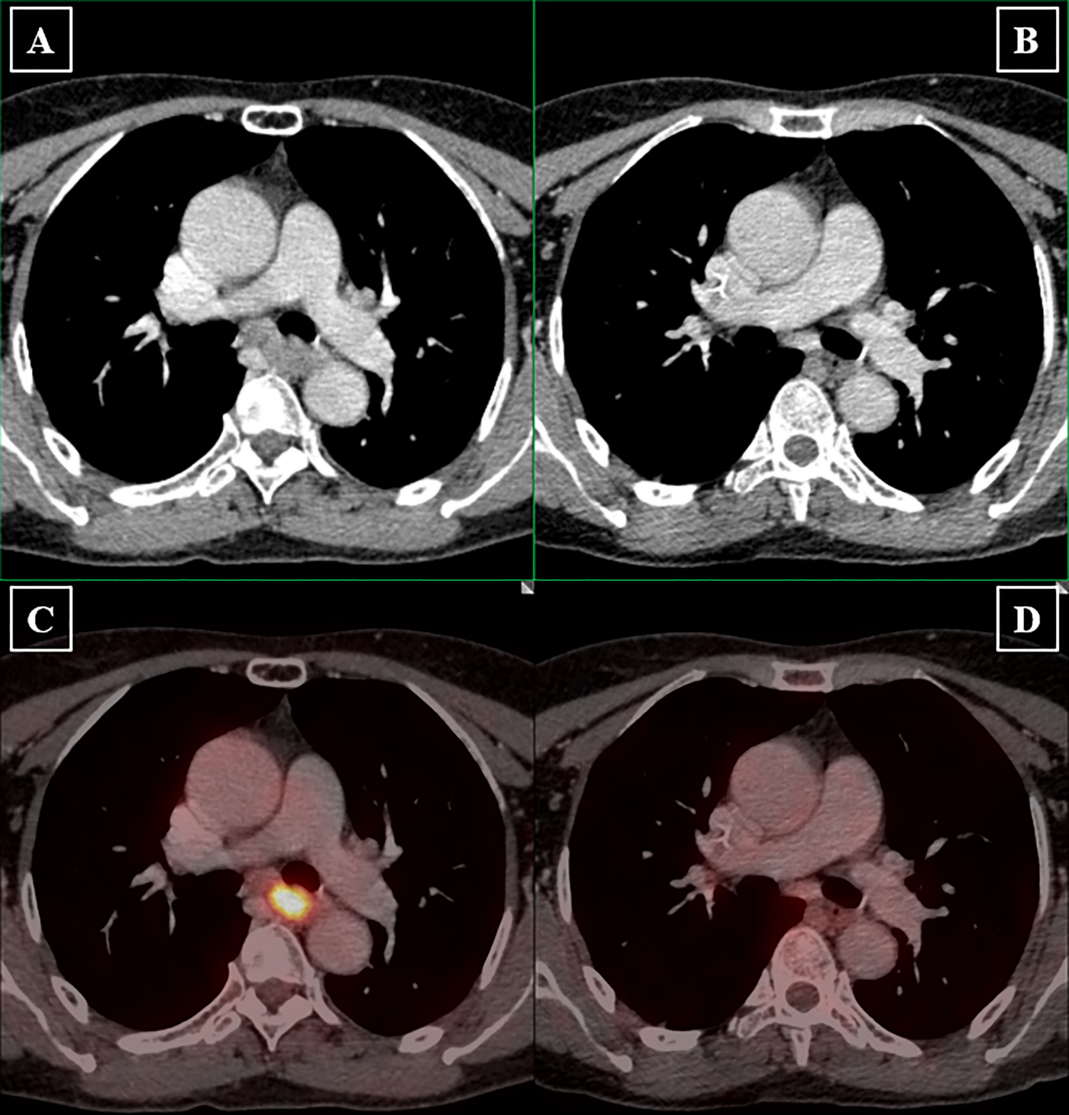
Radiological assessment before and after induction immunochemotherapy. Longest diameters in the plane of measurement of primary lesions were recorded. Lymph nodes were considered malignant if the short axis is longer than 1.5 mm. Pretreatment clinical staging of primary tumor and lymph nodes were determined by both the physician in charge and radiologists. (A) Pretreatment PET-CT image shows that the primary tumor is large, irregular in shape with evident left bronchial compression. The normal esophageal lumen disappears due to extensive thickening of the esophageal wall. (B) Posttreatment PET-CT image shows that significant tumor shrinkage provides clear demarcation between primary tumor and the left bronchus. Esophageal lumen reappears. (C) Pretreatment PET-CT image presents hypermetabolic characteristic of the primary tumor. (D) Subsequent PET-CT image revealed tumor metabolic value reduced to background level.
